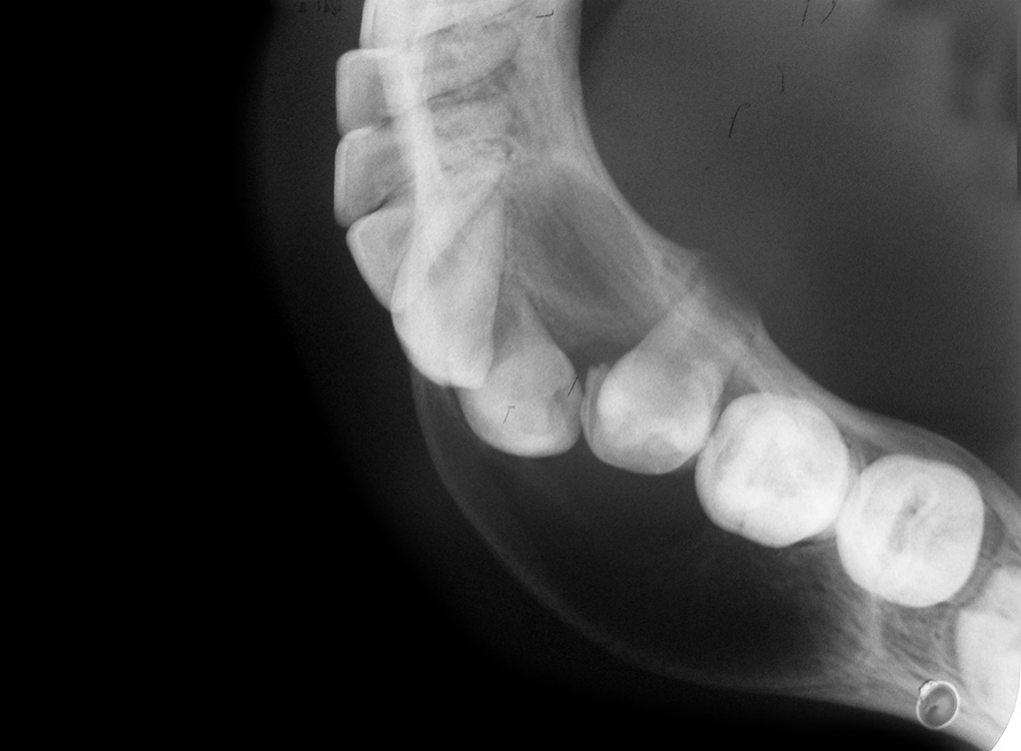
Occlusal radiograph showing buccal cortical plate expansion in relation to 75
